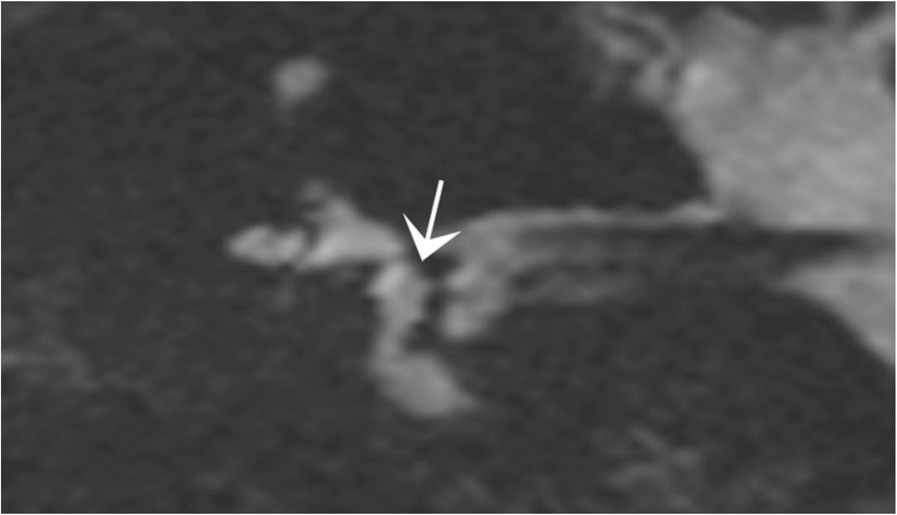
3T MRI. Coronal T2-weighted gradient-echo FIESTA-C in a patient suffering from Meniere disease. The saccule is not clearly visible (arrow), although there are not visible motion artefacts. The absence of visualisation of the saccule can be considered as pathological, as the saccule was always seen in the controls
